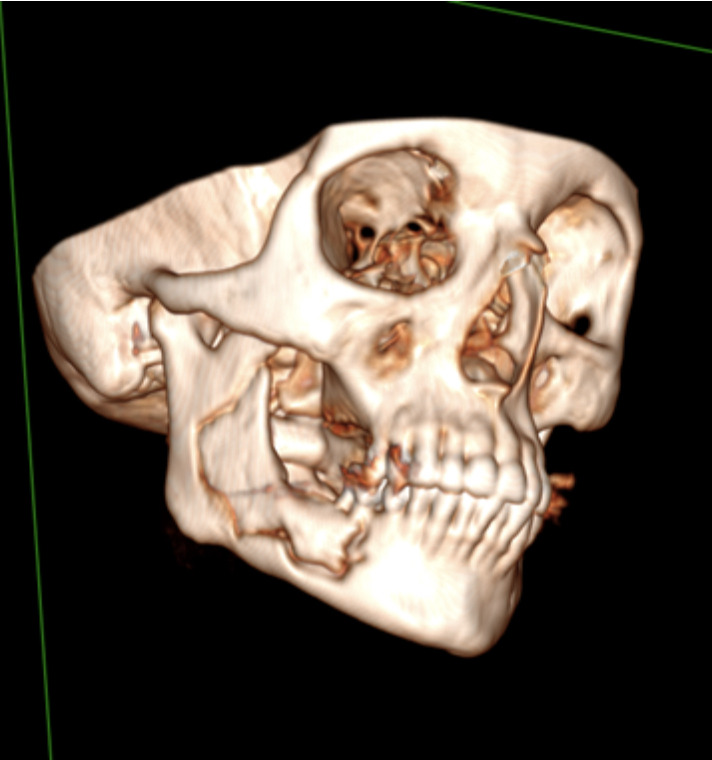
3-D computed tomography reconstruction shows an osteolytic lesion extending from the mandibular body to the right-side ascending ramus.
